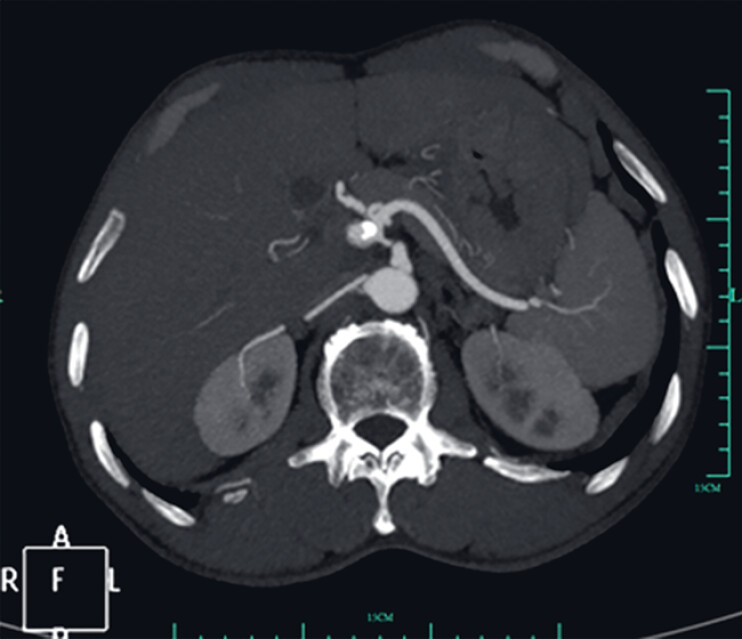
Gastric artery CTA after the first endoscopic ultrasound (EUS) intervention.
